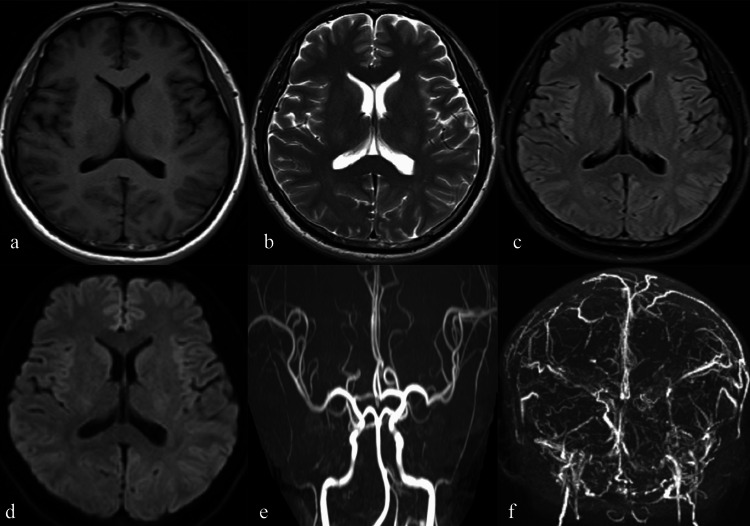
Images of disease recurrence. a-e: MRI and MRA showed unremarkable parenchymal and arterial structures. f: MRV showed thrombosis in bilateral transverse sinus, sigmoid sinus, sinus confluence, superior sagittal sinus and inferior sagittal sinus, and the veins on the brain surface were thickened and tortuous. MRI: magnetic resonance imaging; MRA: magnetic resonance angiography; MRV: magnetic resonance venography
